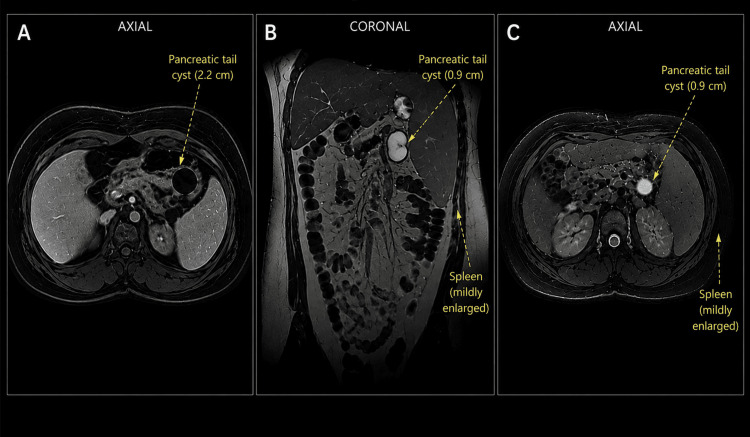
MRI demonstrating the initial pancreatic tail cyst and subsequent interval regression (A) Axial MRI demonstrating a well-circumscribed 2.2-cm cystic lesion in the pancreatic tail without mural nodule, solid component, or restricted diffusion. (B) Coronal follow-up MRI demonstrating interval decrease in cyst size to 9 × 8 mm with mild splenomegaly. (C) Axial follow-up MRI confirming interval regression of the pancreatic tail cyst and persistent mild splenomegaly. No abnormal enhancement, solid components, or restricted diffusion were identified on follow-up imaging. MRI: magnetic resonance imaging
